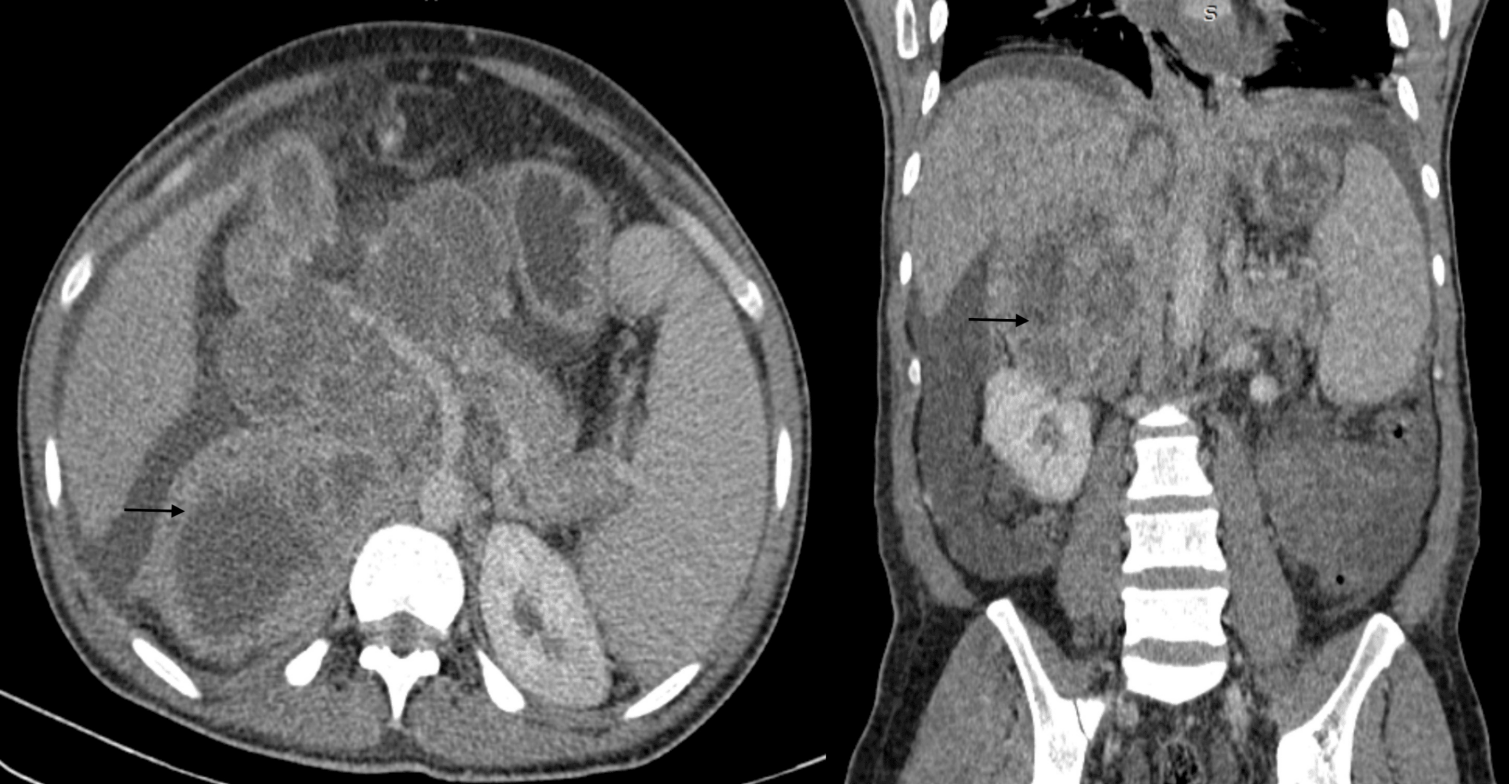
Post-contrast CT showing heterogenous enhancement of the large right adrenal mass (black arrows)
